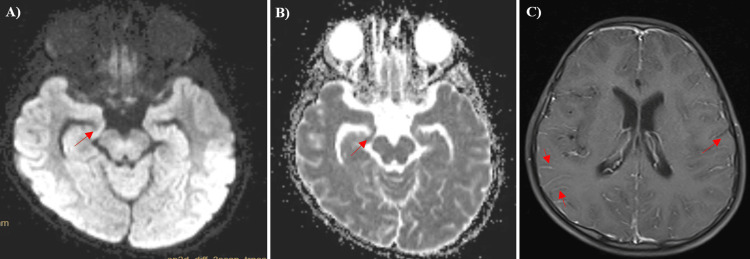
Images A and B - MRI brain showing diffusion restriction (arrow) in DWI (diffusion-weighted image) and ADC (apparent diffusion coefficient) sequences in the bilateral hippocampal area; Image C - MRI brain T1 sequence contrast image - showing leptomeningeal enhancement (arrows).
